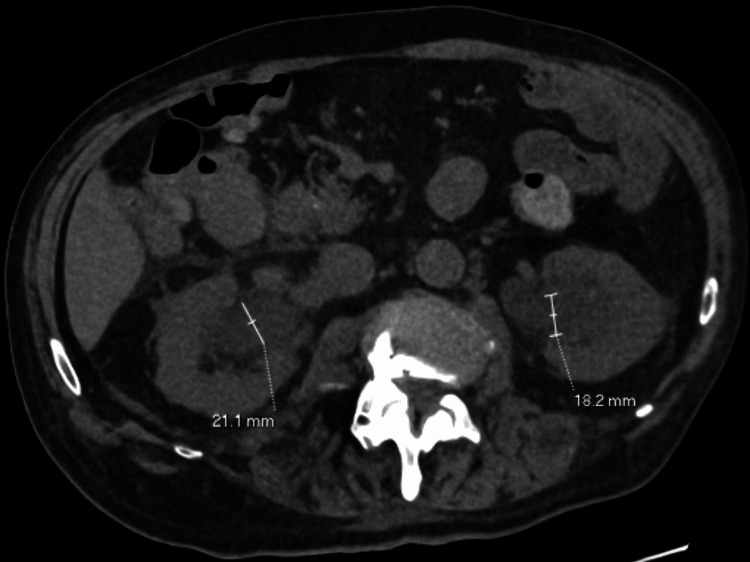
Axial view of the CT scan of the abdomen and pelvis showing moderate to severe bilateral hydronephrosis
